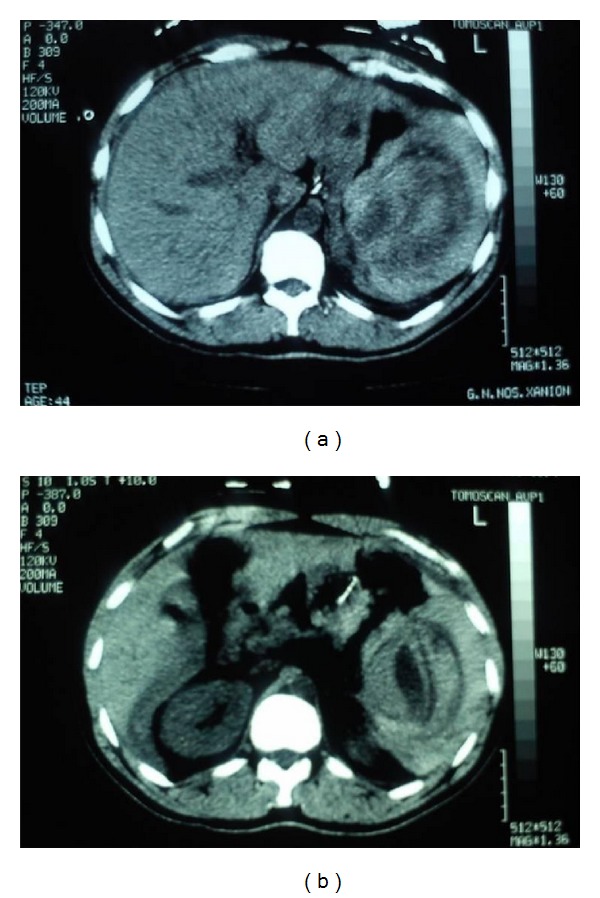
Axial computed tomography of the abdomen showing a ruptured spleen and haemoperitoneum.
